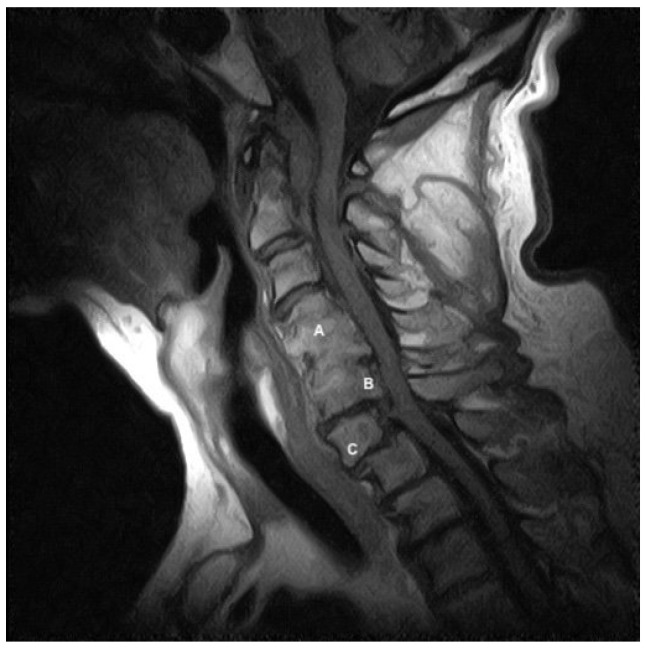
Sagittal T1-weighted MRI image reveals a synostosis at C4-5 (A), a retrolisthesis of C6-7 (B), and an anterolisthesis of C7-T1 (C).
